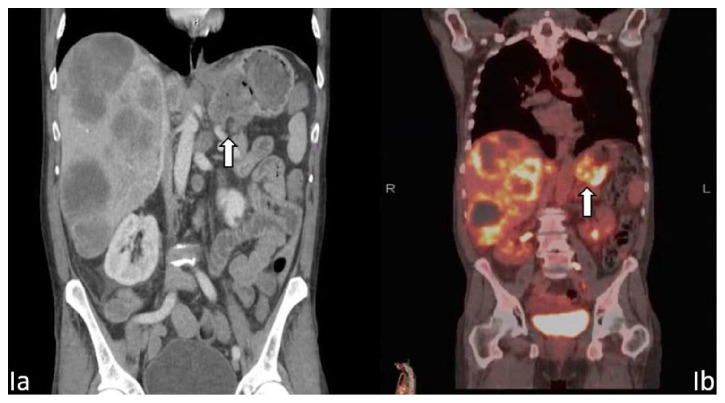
(a) CT demonstrating gastric mass in the lesser curvature (arrow) with diffuse hepatic metastases. (b) PET scan with 6.5 cm hypermetabolic gastric (Arrow) and no additional sources for a primary tumor. Diffuse hepatic metastases again noted.
